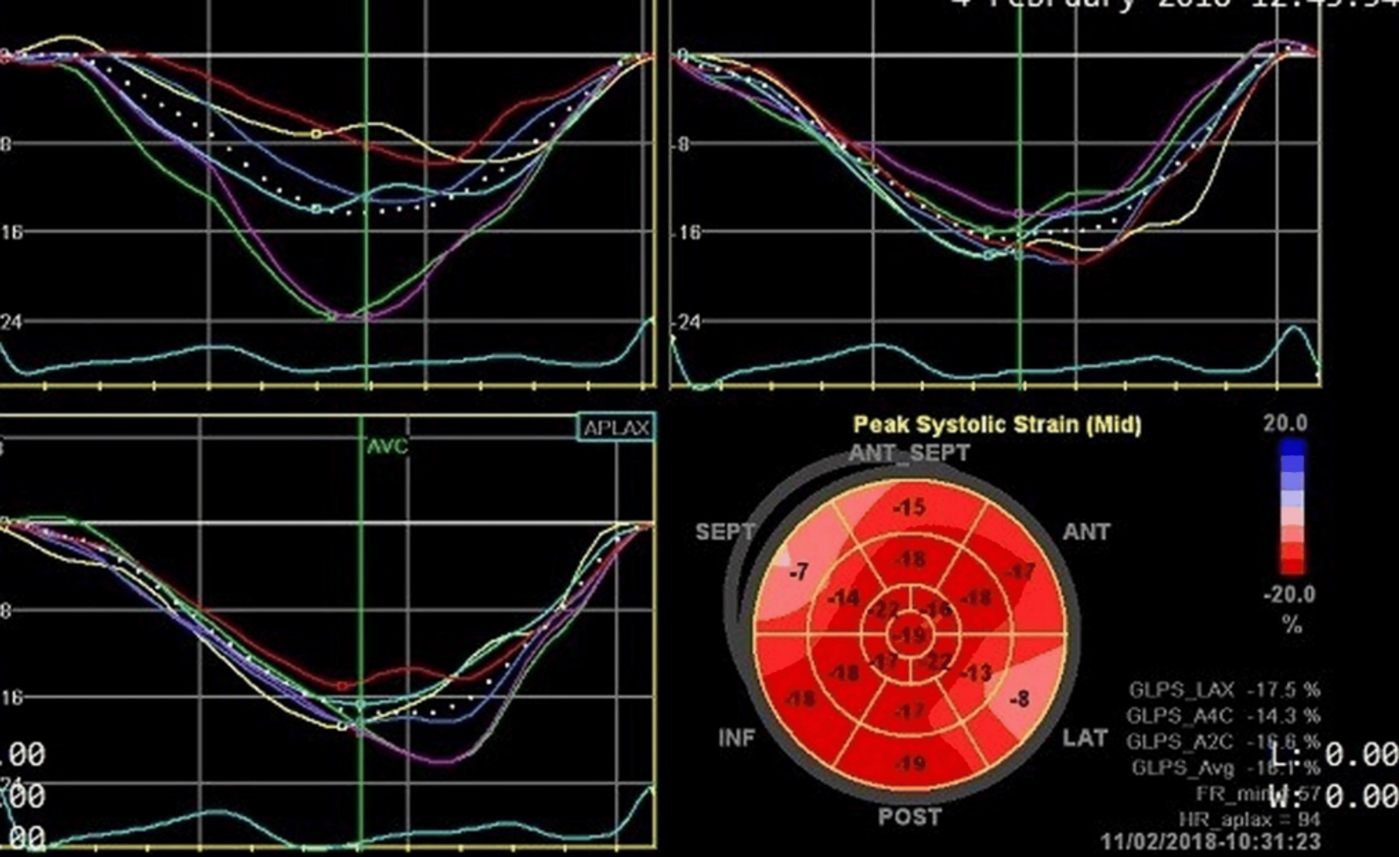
Measurement of LV GLS Speckle-tracking echocardiography was used to analyze LV GLS at frame rates greater than 50 s⁻¹. The peak longitudinal strains from each of the 17 LV segments, whether negative or positive, were automatically averaged from the apical four-chamber, two-chamber, and long-axis views to calculate LV GLS. GLS = global longitudinal strain; LV = left ventricular
